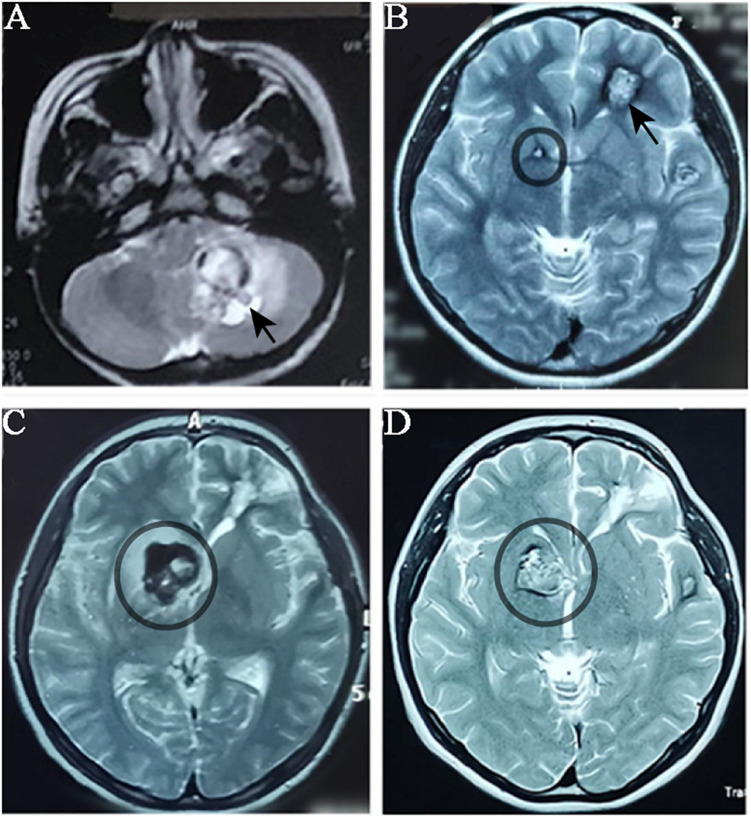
MRI imagology of the proband’s head during the progression of CCMs. The proband had CCMs-associated haemorrhage for four times over five years. MRI images were taken when the proband patient had haemorrhage. (A) MRI (T1-weighted) detected a lesion located in the left cerebellar hemisphere (black arrow) when the proband had her first haemorrhage. (B) When the proband had her second haemorrhage, MRI (T2 weighted) showed a lesion in the left frontal lobe (which was surgically removed) and a small lesion in right basal ganglia (black circle). (C) and (D) The lesion in right basal ganglia was obviously enlarged in two years.
